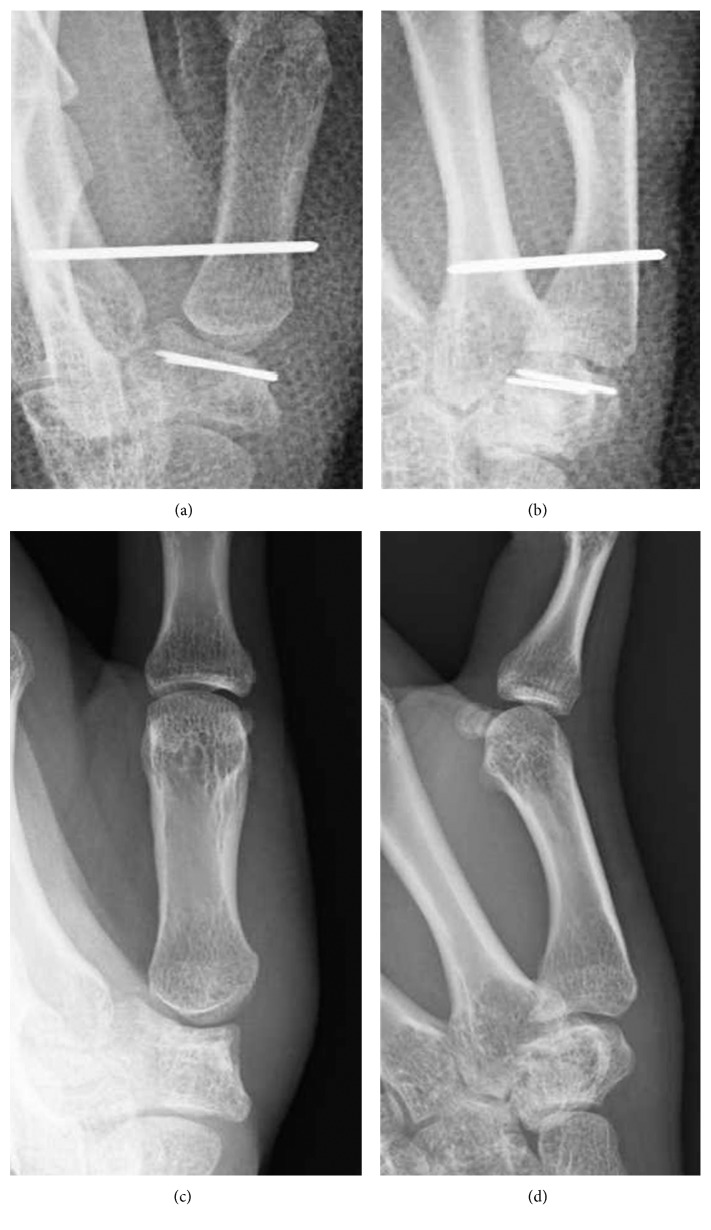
(a, b) Radiographs taken directly after surgery. The collapsed articular surface was elevated, and *β*-TCP was used to fill the subchondral bone loss, fixed by K-wire. (c, d) Radiographs taken at final follow-up, 2 years after surgery. A congruent CMC joint and trapezium fracture union is shown; however, a hyperextension of the thumb metacarpophalangeal (MP) joint was revealed. (a, c: anteroposterior view; b, d: lateral view.)
